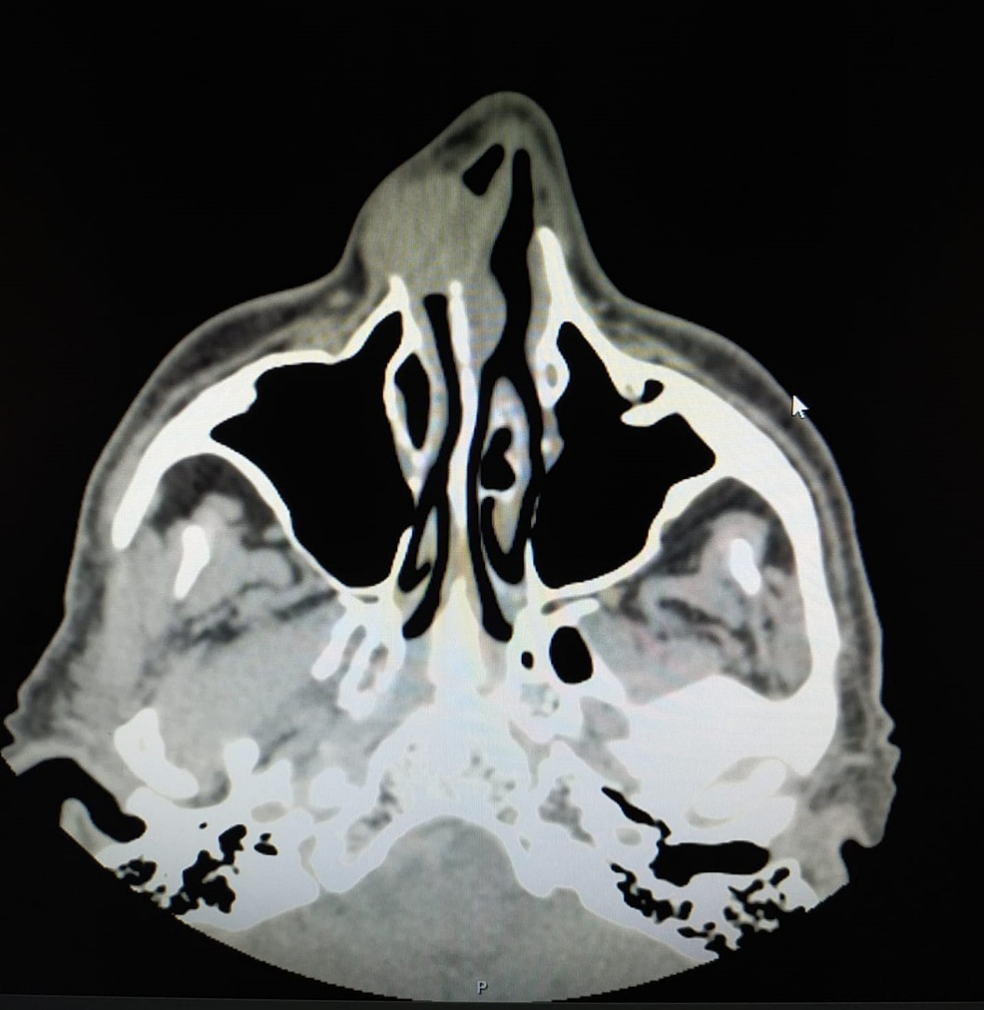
CT scan - axial view CT scan showing a homogeneous nodular mass of soft tissue with the erosion of the frontal maxillary apophysis extending into the nasal vestibule CT: computed tomography
